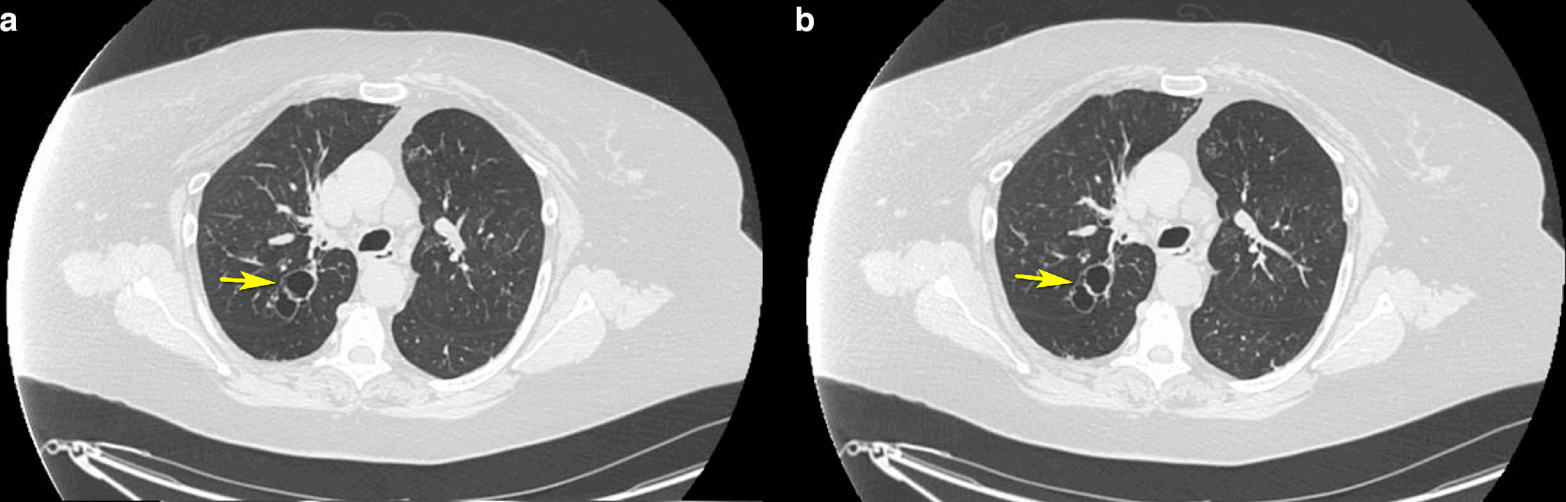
A and B. Axial non-contrast CT scan of the chest in inspiratory phase demonstrating hyperinflation of both lungs with multiple ballooning cystic bronchiectasis distal to third-generation bronchi bilaterally.
